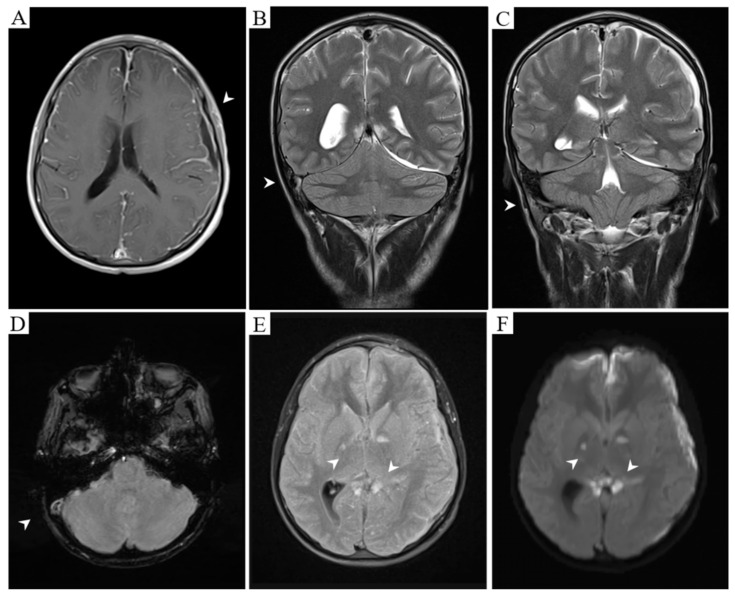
Meningeal enhancement and left fronto-temporo-parietal empyema. Empty delta sign is also observed (A, T1 post-gadolinium). Right transverse (B, T2) and sigmoid (C, T2) sinuses occlusion due to an acute thrombus (D, Gradient echo). Established ischemia affecting bilateral globus pallidus and corpus callosum splenium (E, T2 FLAIR) with diffusion restriction (F, DWI).
